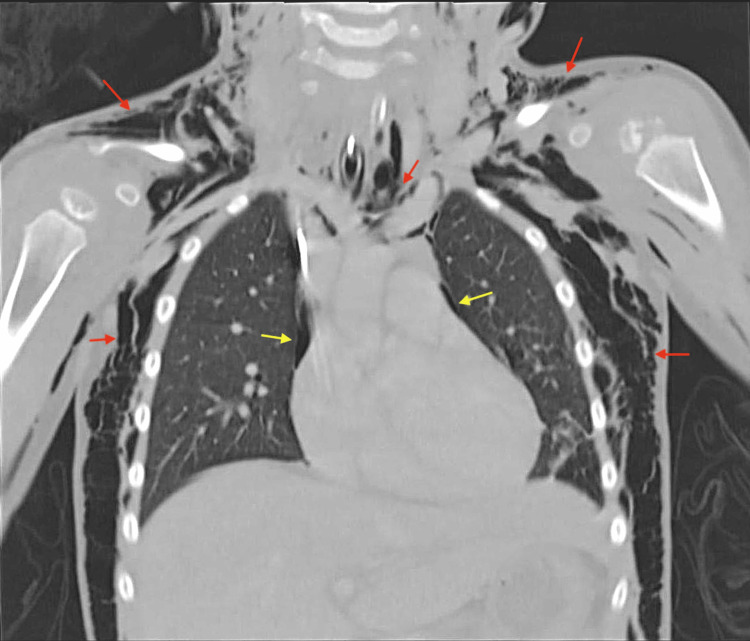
Coronal CT Chest showing diffuse surgical emphysema and bilateral small pneumothoraces Immediately postoperative CT demonstrating extensive diffuse surgical emphysema (red arrows) involving the neck, upper thorax, and bilateral upper limbs. Multiple small bilateral pneumothoraces (yellow arrows) are also visible. The image highlights the widespread air tracking through the subcutaneous and fascial planes.
